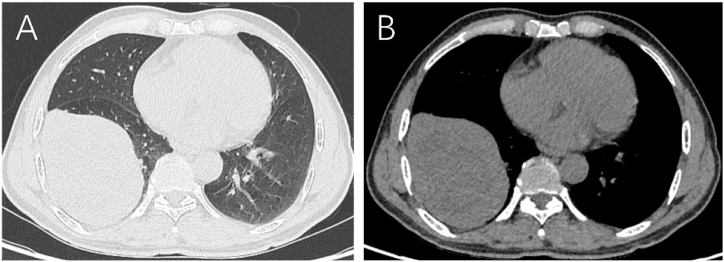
Computed tomography (CT) revealed an 11-cm mass in the right lower lobe of the lung to have heterogeneous density, relatively clear margins, and no calcification. A (Lung Window), B (Mediastinal Window).
